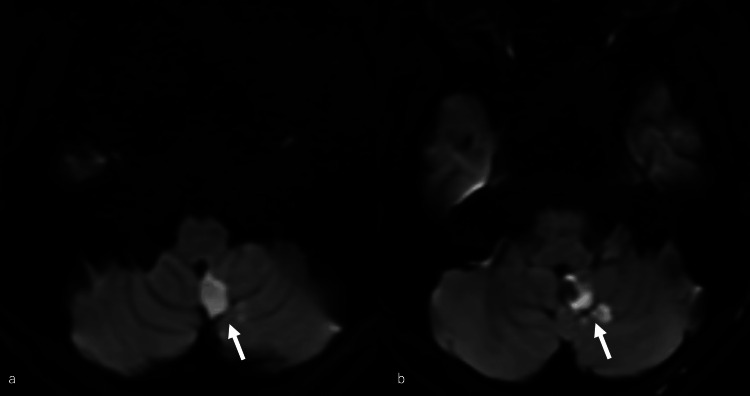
Magnetic resonance imaging performed the day after surgery revealing a cerebellar infarction (white arrow)
